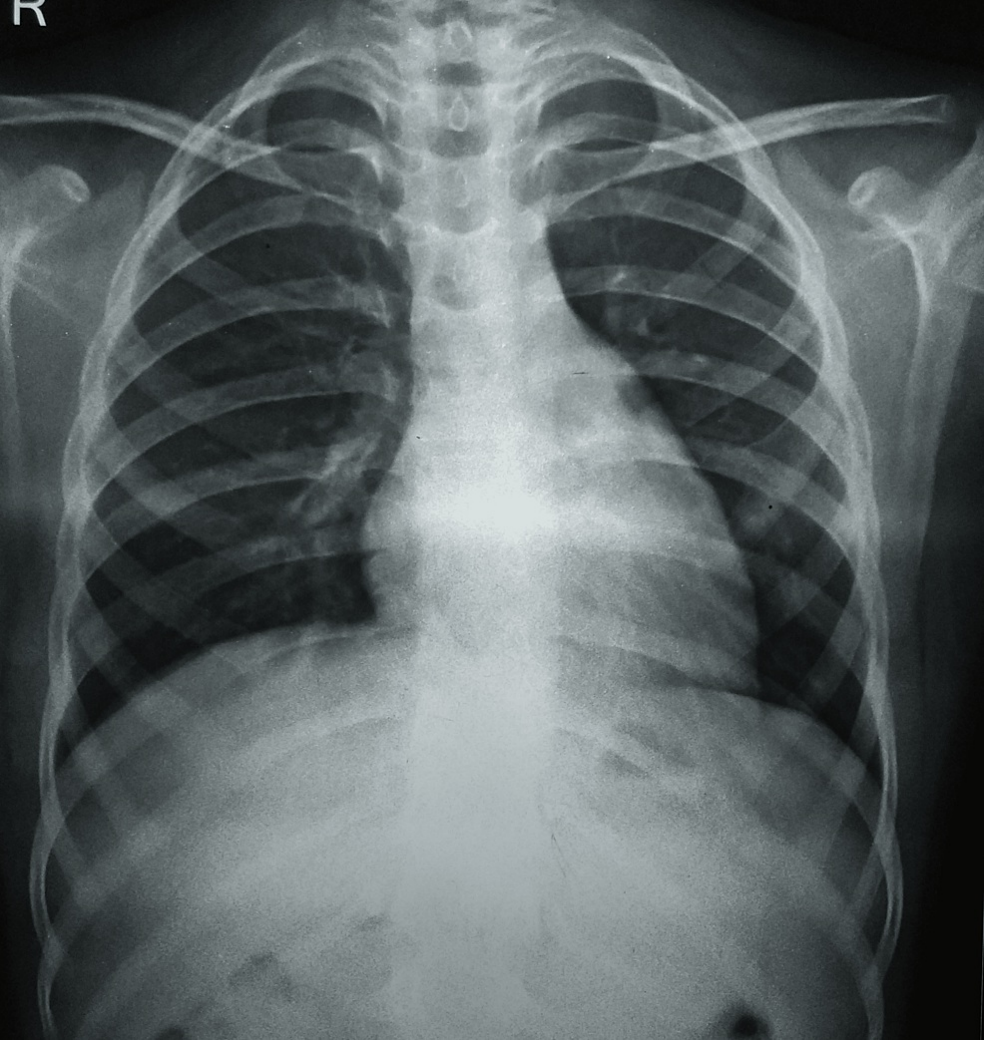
A normal chest radiograph, posteroanterior view
